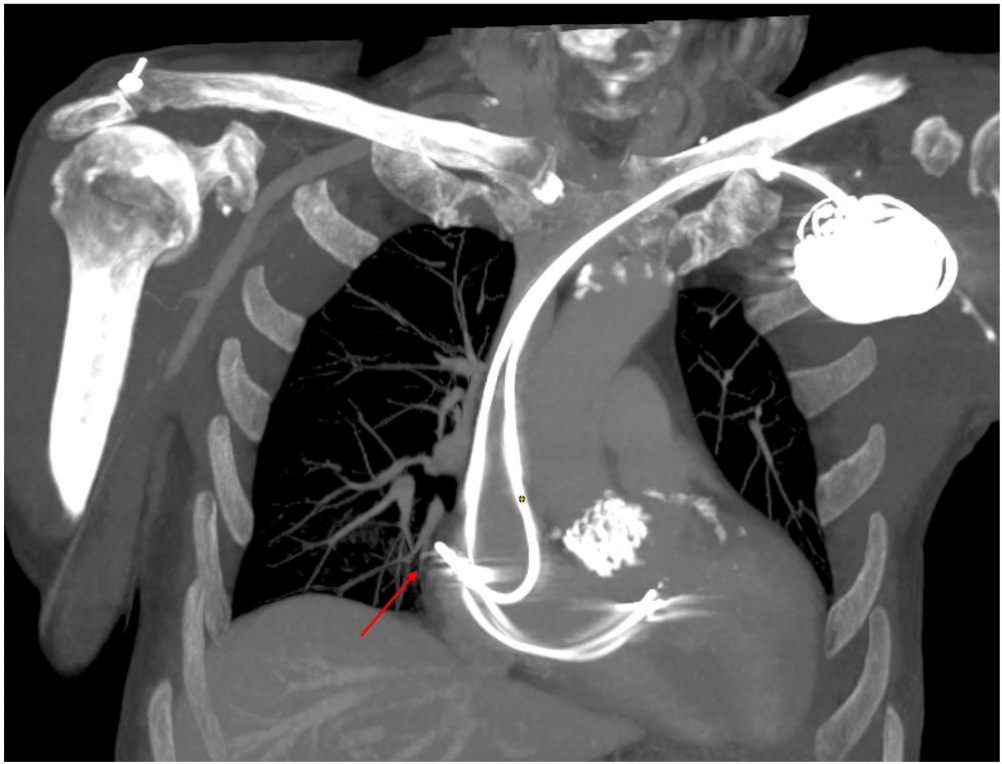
Chest computed tomography scan 6 h post-implantation revealing perforation of the atrial lead through the right atrial wall, in contact with the right pulmonary venous tree, without contrast extravasation (arrow).
